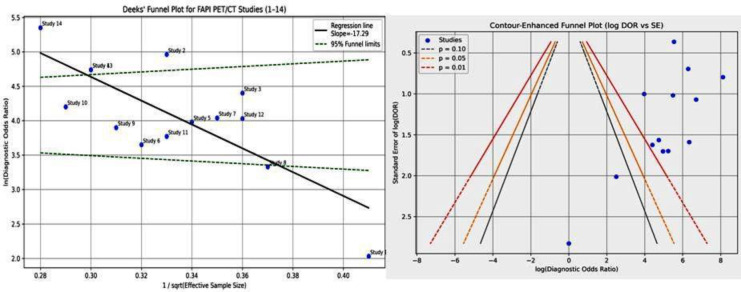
A) Deek’s Funnel plot: Four studies are seen falling outside the 95% funnel, however, Deeks’ P-value is 0.949, hence the observed regression slope (–17.29) is not statistically different from zero. A high P-value confirms no formal evidence of significant small-study effects or publication bias in FAPI PET/CT dataset. B) Contour enhanced funnel plot showed visual asymmetry, with a relative paucity of studies in the lower-left region marked asymmetry, with an excess of highly significant DOR estimates and a dearth of null results. This pattern is consistent with publication bias and small-study effects rather than random variation (small studies with low log[DOR]). Trim and Fall analysis was used for correction of this bias in the contour plot
